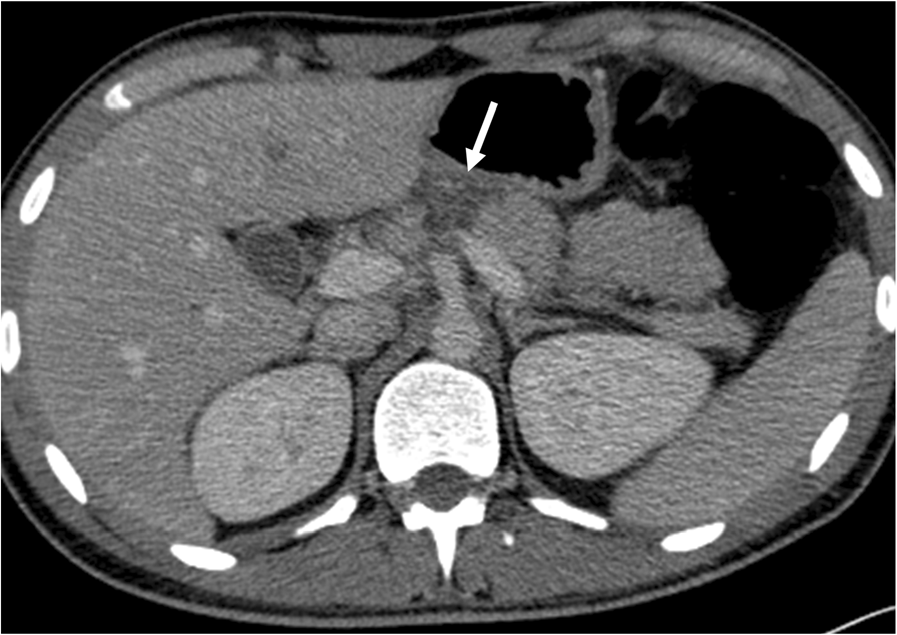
Contrast-enhanced computed tomography of the abdomen reveals a full thickness laceration of the pancreatic neck (arrow) with a lesser sac fluid collection (not shown), suggesting pancreatic duct disruption.
